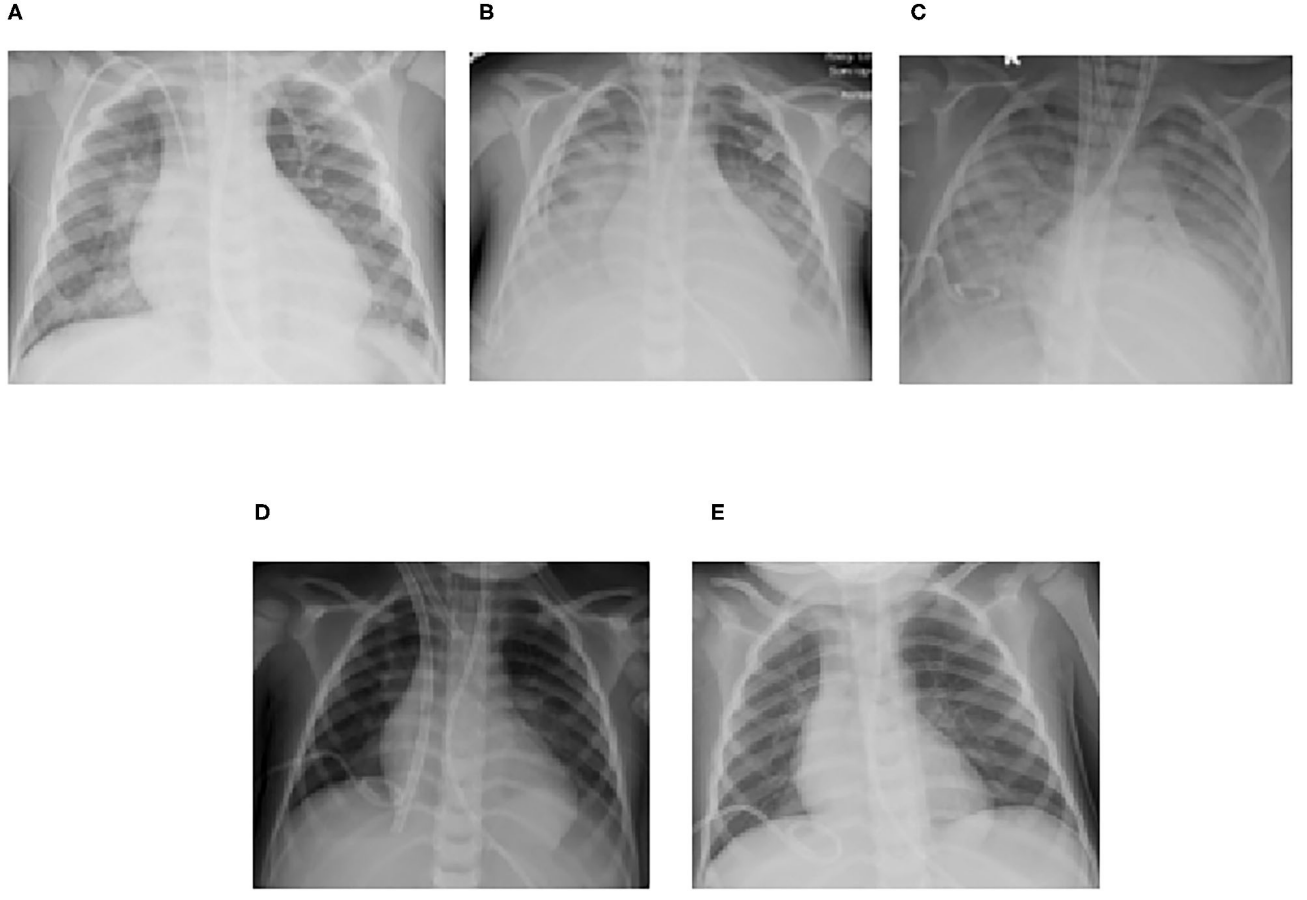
Serial chest radiographs for Case 2 demonstrating the progression and subsequent improvement of diffuse bilateral interstitial and alveolar opacities (A) Following intubation, (B) Prior to transfer for ECMO evaluation, (C) Following ECMO cannulation, (D) Prior to ECMO decannulation, (E) Post-transfer back to referring center.
